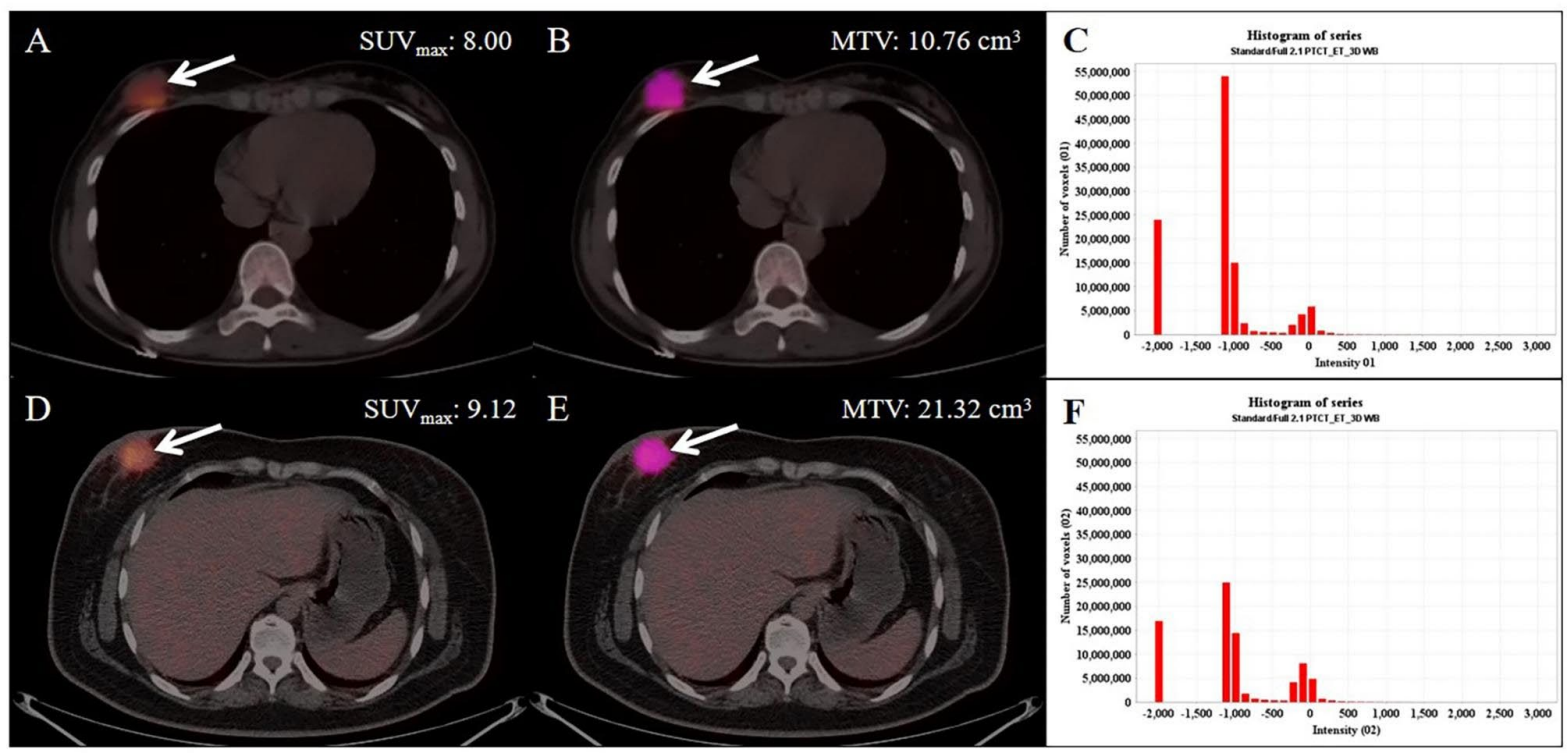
A typical case of the relationship between MTV and AR expression. Two patients were diagnosed with IDC (invasive ductal breast carcinoma) successively in a year. The former (A: PET/CT fusion image, B: The VOI of the lesion, C: The histogram of VOI) was confirmed as AR-positive (SUV_max_: 8.00; MTV: 10.76 cm^3^). The latter (D: PET/CT fusion image, E: The VOI of the lesion, F: The histogram of VOI) was confirmed as AR negative (SUV_max_: 9.12; MTV: 21.32 cm^3^). These lesions were similar on PET/CT images but showed significant differences in the histograms of the radiomic features
